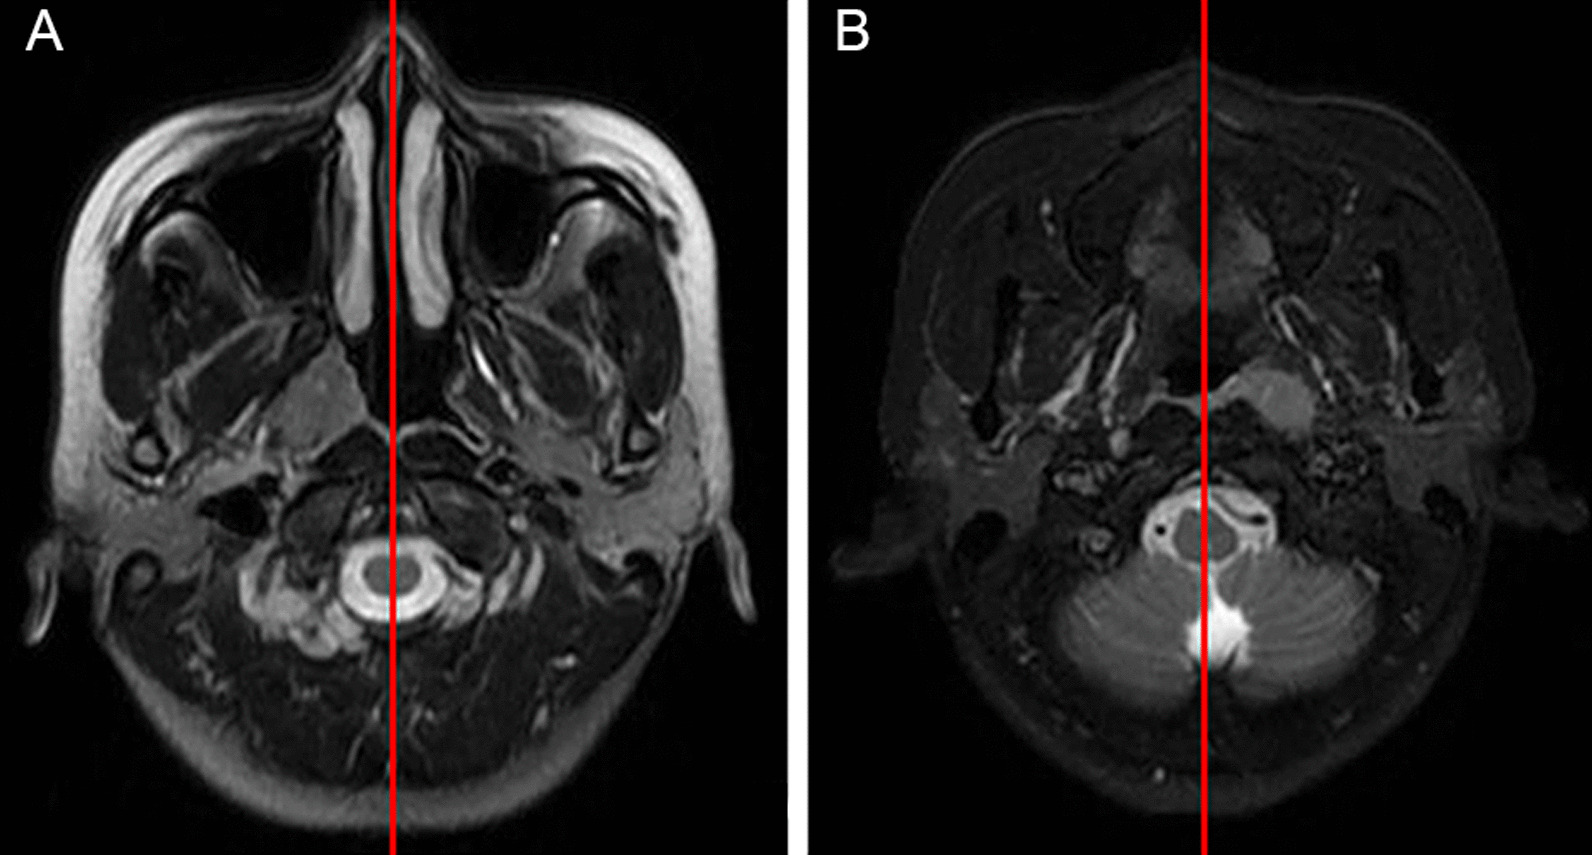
Criteria for diagnosis of unilateral nasopharyngeal carcinoma: A MRI of a lesion that did not exceed the midline of the nasopharynx; B MRI of a lesion that reached the midline but did not exceed the midline
